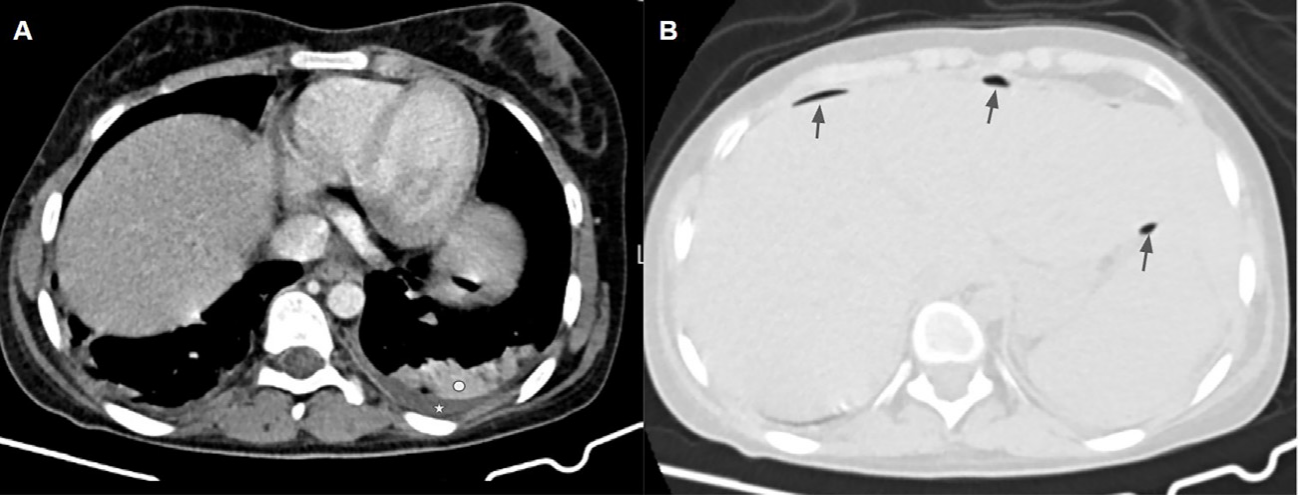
(A) Chest CT scan-Mediastinal window shows left-sided pleural effusion (white star) with adjacent subsegmental consolidation (white circle) in the posterobasal aspect of the left lower lobe. (B) Abdominal CT scan-pulmonary window depicts mild pneumoperitoneum (gray arrows) adjacent to the anterior surface of the liver and spleen.
